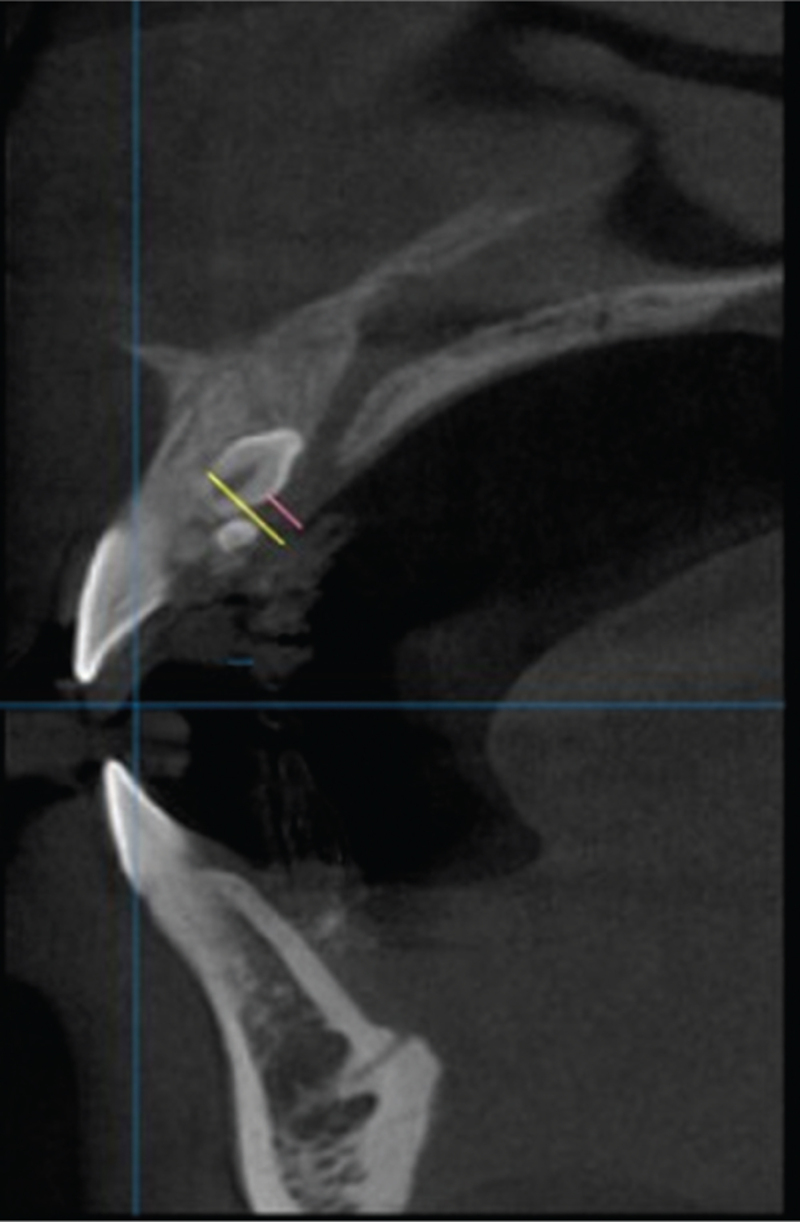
Cone beam computed tomography image of the patient's mouth in sagittal view. The yellow line marks the distance from the supernumerary tooth's labial margin to the palatal mucosa (L1). The yellow line marks the distance from its palatal margin to the mucosa (L2).
